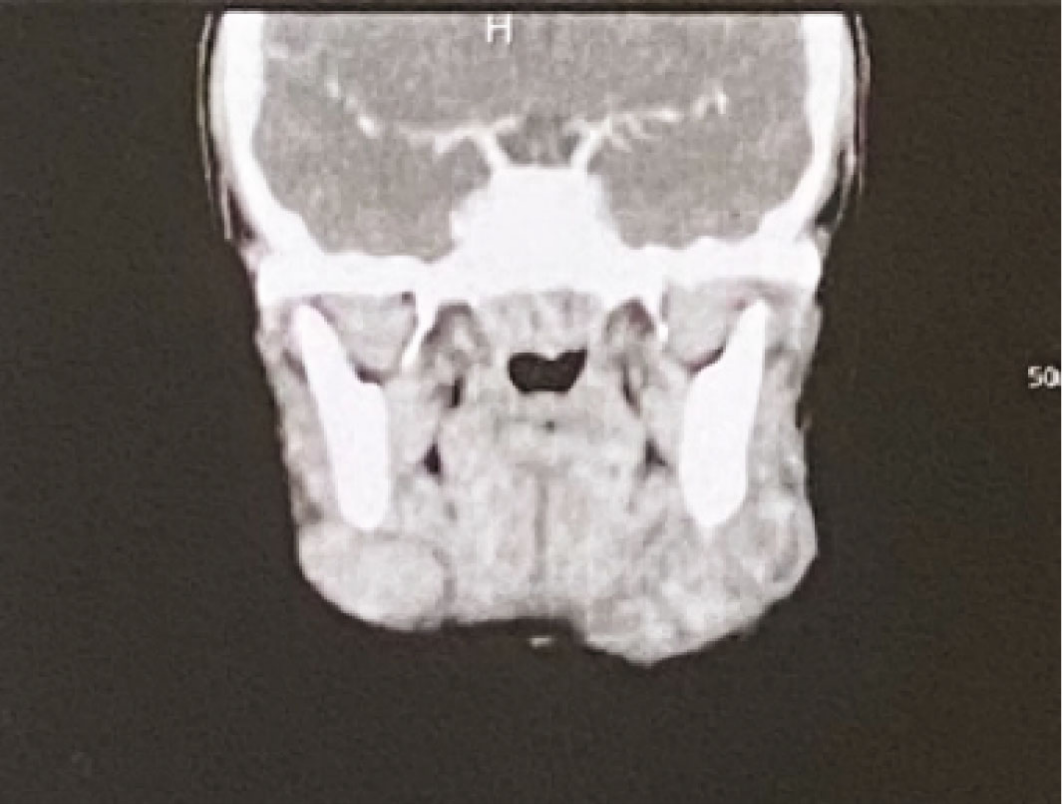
Computed tomography scan of the neck showing enlarged bilateral lymph nodes. On the patient's left, the largest lymph node has a central hypodensity consistent with necrosis.
